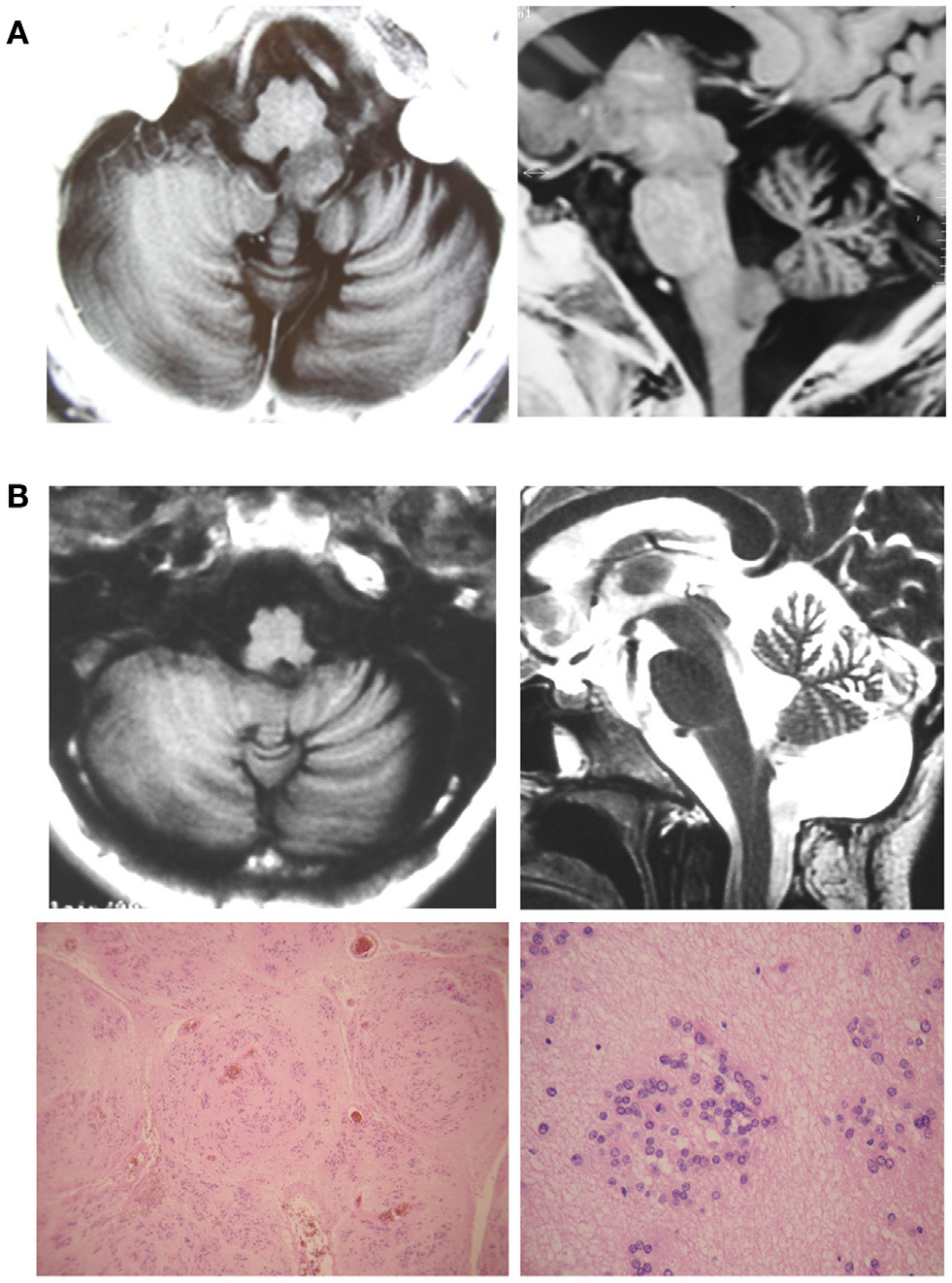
(A) Case 1. Preoperative T1-weighted brain MRI scan showed a hypointense and homogenous mass within the left MO without Gadolinium enhancement. (B) The postoperative T1-weighted brain MRI scan showed complete resection of the tumor.
